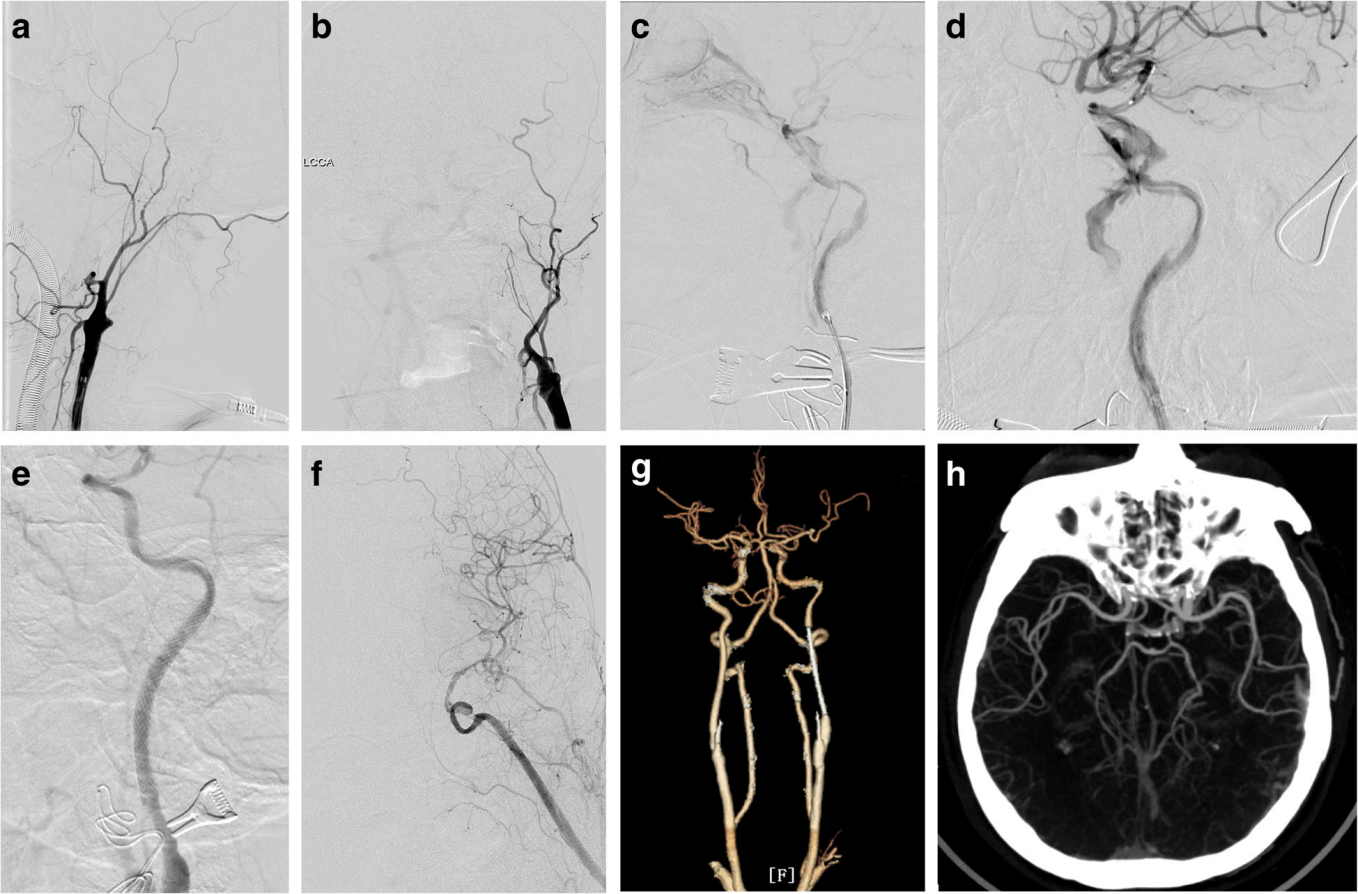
Case example. Case 4 of hybrid surgery for a symptomatic chronic internal carotid artery occlusion (ICAO) and a new development of intraprocedural carotid-cavernous fistula (CCF). a, b Left ICAO, revealed by preoperative angiographies on lateral and posterior-anterior projection, respectively. c A new development of intermediate-flow CCF with anterior and inferior drainage, along with spiral and cervical-to-cavernous ICA dissection after surgical removal of the ICAO plaque, revealed by angiographies via PLUS microcatheter (Codman & Shurtleff, Raynham, MA). d Angiography via the guiding catheter following negotiation of the assembly of microcatheter and microwire into normal ICA distal to the dissection. e, f Successful ICA recanalization and complete CCF obliteration after telescopically deploying one 7 × 40 mm Wallstent (Boston Scientific Corporation, Natick, MA) and two 4.5 × 37 mm Enterprise stents through the true channel of the dissection. g, h Durable patency of the left ICA and complete CCF obliteration on follow-up CT angiogram at 6 months
